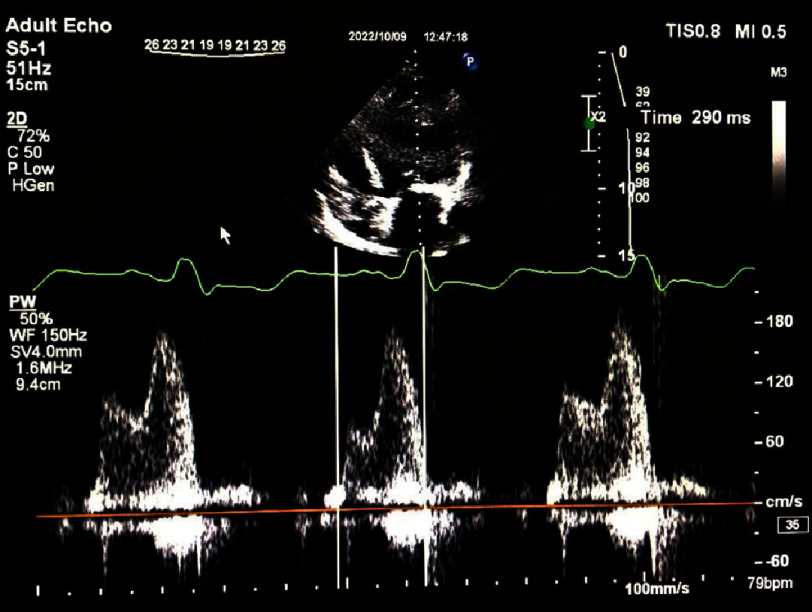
An apical four-chamber view (pulsed doppler recording) showing LV filling time =290 ms.
